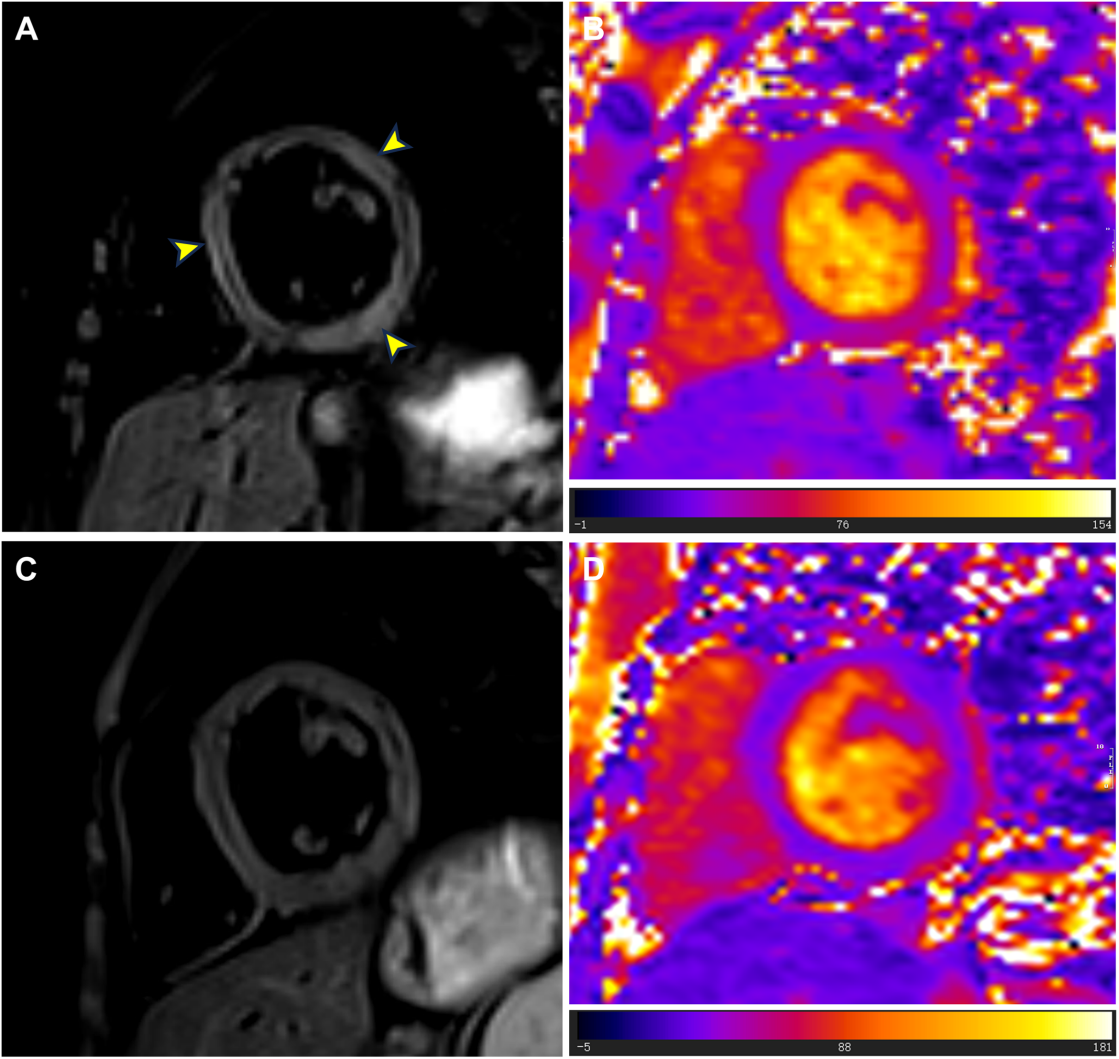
T2-weighted imaging and T2 mapping images at admission and follow-up. (A) At admission, T2-weighted imaging showed localized high signals in the left ventricular septum, lateral wall, and inferior wall (yellow arrowheads). (B) At admission, T2 mapping images showed abnormally elevated T2 values (mean value = 56.9 ms) in the basal to middle segments of the interventricular septum and inferolateral walls. (C) During the follow-up cardiac magnetic resonance examination in July 2024, T2-weighted imaging showed no signs of myocardial edema. (D) During the follow-up cardiac magnetic resonance examination in July 2024, T2 mapping images showed reduced T2 values (mean value = 42.0 ms) in the basal to middle septal and inferolateral walls.
